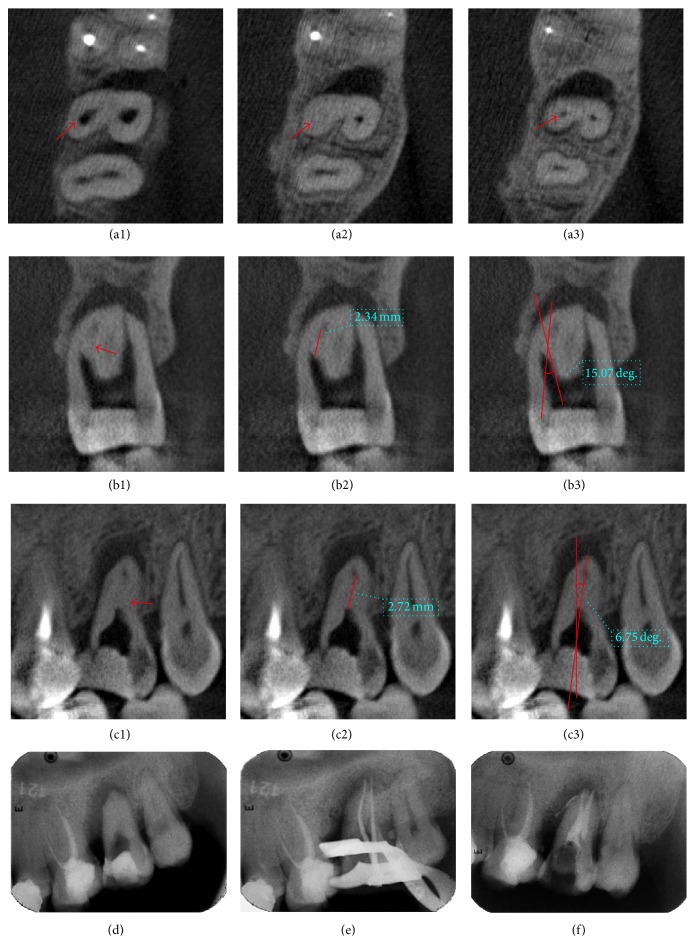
Case  2: (a1) upper part of the palatal canal over calcification managed by ultrasonic instrument before CBCT scanning (red arrow). (a2) Calcification of palatal canal (red arrow). (a3) Canal under calcification (red arrow). (b1)–(b3) Determination of depth in buccolingual direction and BLIA. (c1)–(c3) Determination of depth in mesiodistal direction and MDIA. (d) Radiograph before operation. (e) Affirmation of working length. (f) Canals after filling and temporary restoration.
